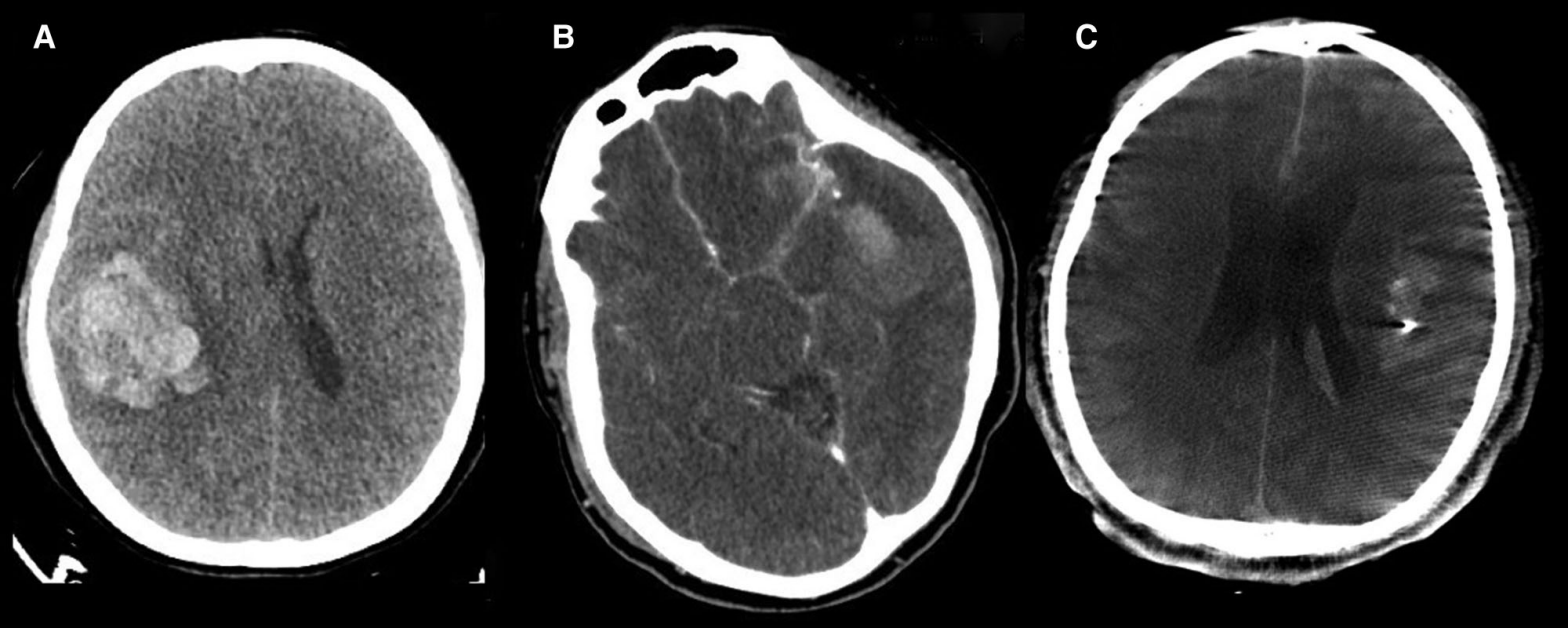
Image parameters documented on the initial brain CTA. (A) Distal hematoma. (B) Perisylvian low density. (C) CTA spot signs.
